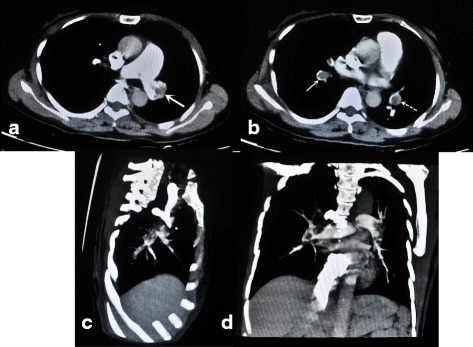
a show the thrombosis at left main pulmonary artery in chest CT angiography. b show the obstruction in both pulmonary arteries (solid arrow:right side, dotted arrow:left side). Extensive obstruction in both pulmonary arteries were showed in lateral view(c) and frontal view (d) of chest CT angiography
